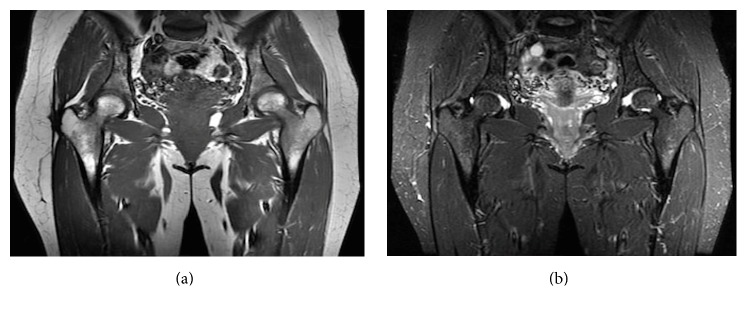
(a) Coronal T1 MRI. (b) Coronal T2 MRI.
